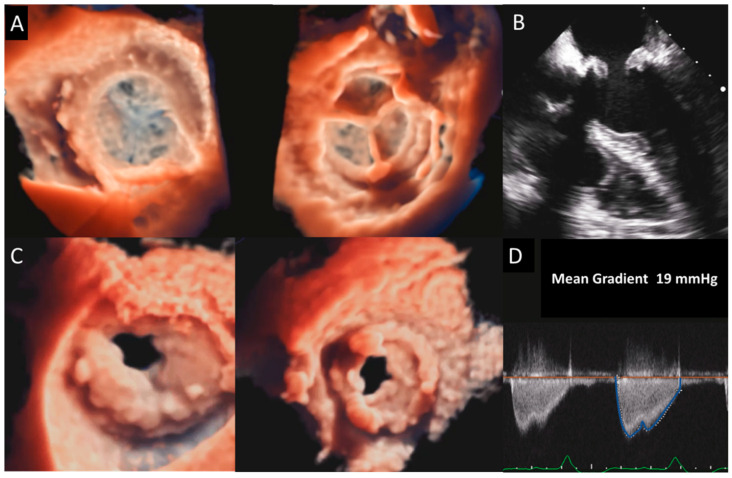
Biological mitral valve thrombosis. (A) 3D TEE appearance of the LA and LV of a normal MV prosthesis compared with (C) a thrombosis of an MV prosthesis. Thickening and obstruction of the valve are clearly shown by 3D TEE imaging and confirmed by thickening and thrombosis at 2D TEE (B) associated with a marked increase in the mean diastolic gradient (D). MV, mitral valve; TEE, transesophageal echocardiography.
